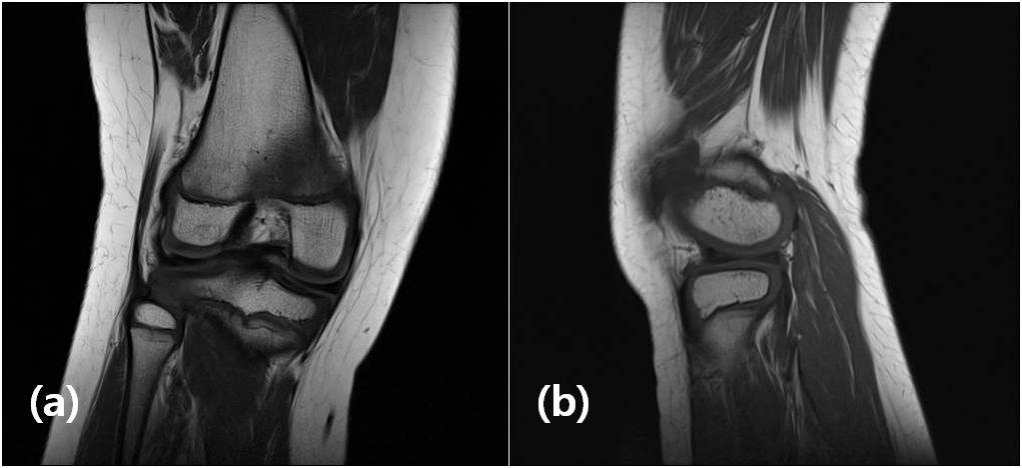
Our patient five months after operation. MRI study showing complete healing of the osteochondritis dissecans lesion of the lateral femoral condyle.
